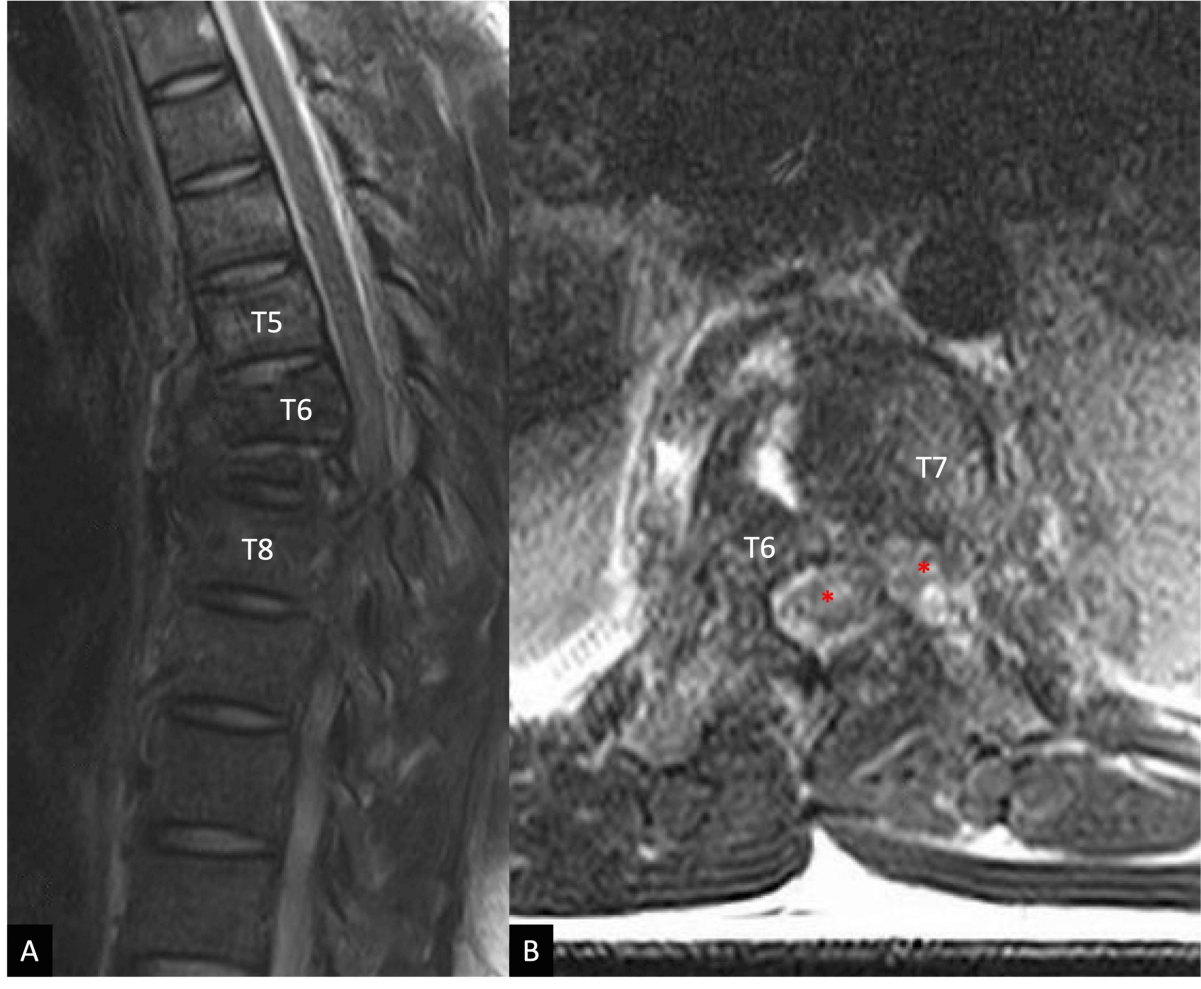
Pre-operative T2-weighted magnetic resonance imaging of the thoracic spine. (A) Sagittal magnetic resonance imaging demonstrating signal changes at the level of the traumatic injury. (B) Axial magnetic resonance imaging demonstrating a dual spinal canal with neural elements, consistent with stretch and/or transection injury to the spinal cord. Two red asterisks label each component of the dual spinal canal. T6 and T7 indicate thoracic vertebral levels.
